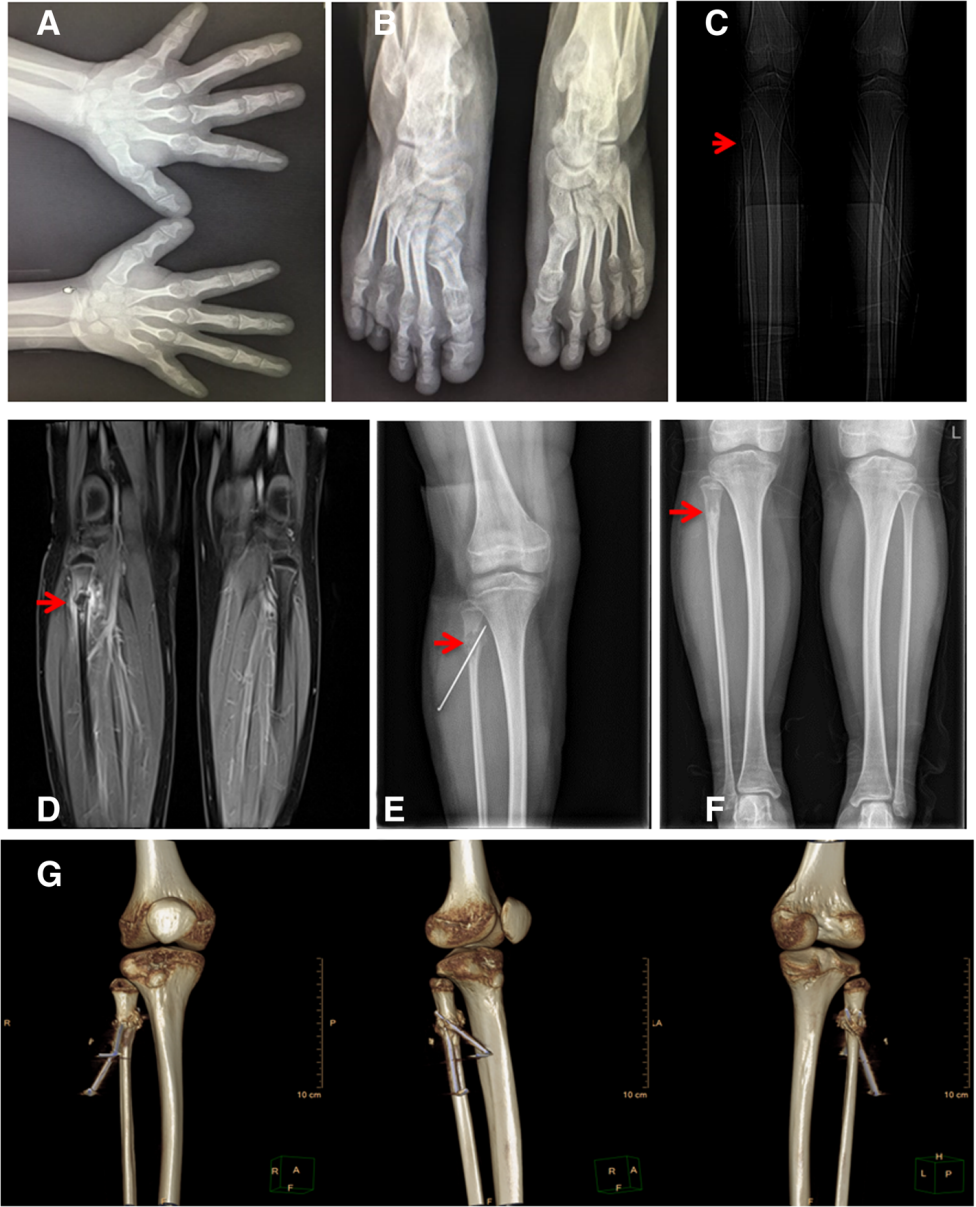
Radiography of the girl. a: Hands; b: Feet; c: Tibiofibular X-ray before the surgery; d: Fibula MRI; e: Tibiofibular X-ray 1 month after surgery; f: Tibiofibular X-ray 10 months after surgery; g: Tibiofibular three-dimensional CT 1 month after surgery
